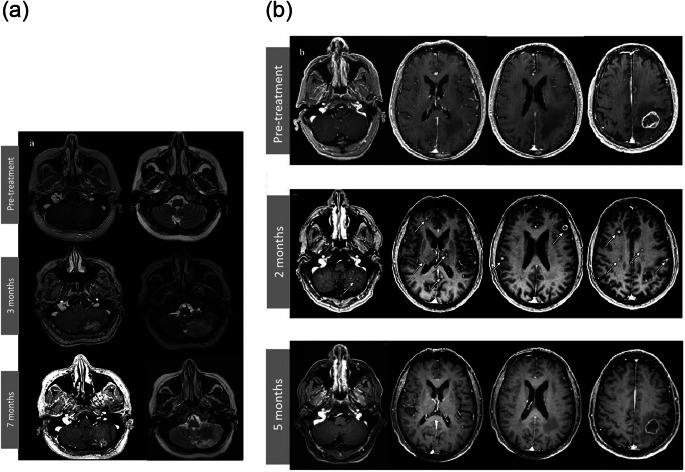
a: Post-contrast T1- and T2-weighted images in a patient with a left cerebellar metastatic lesion taken before treatment initiation, after 3 months of ICI treatment, and after 7 months of ICI treatment. Brain MRI after 3 months of treatment showed increased contrast enhancement and edema, which spontaneously improved 4 months later. b: Post-contrast T1 weighted images in a patient with an initial left parietal metastatic lesion taken before treatment initiation, after 2 months of ICI treatment, and after 5 months of ICI treatment. Brain MRI after 2 months of treatment showed multiple new disseminated lesions (white arrows), which spontaneously disappeared 3 months later
